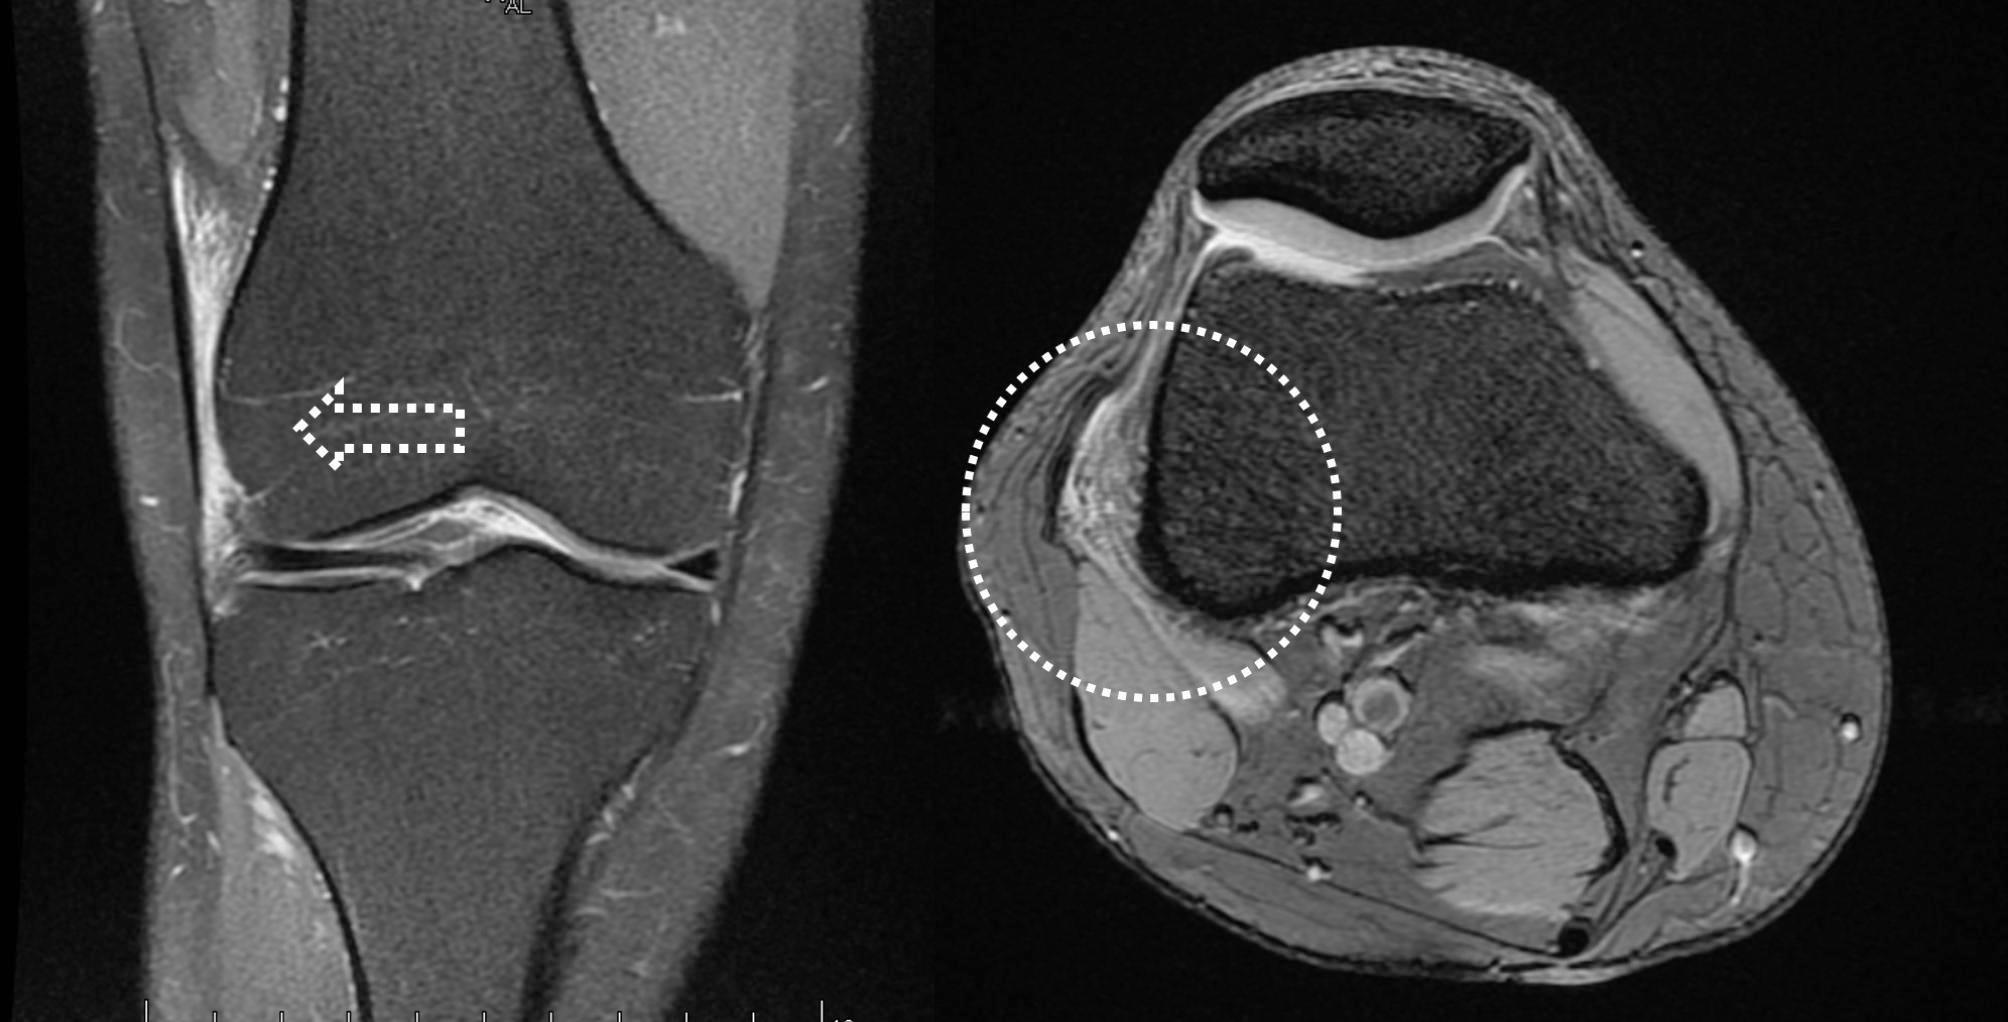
Preoperative MRI: coronal (right) and axial (left) views showing edema at the level of the ITB.
